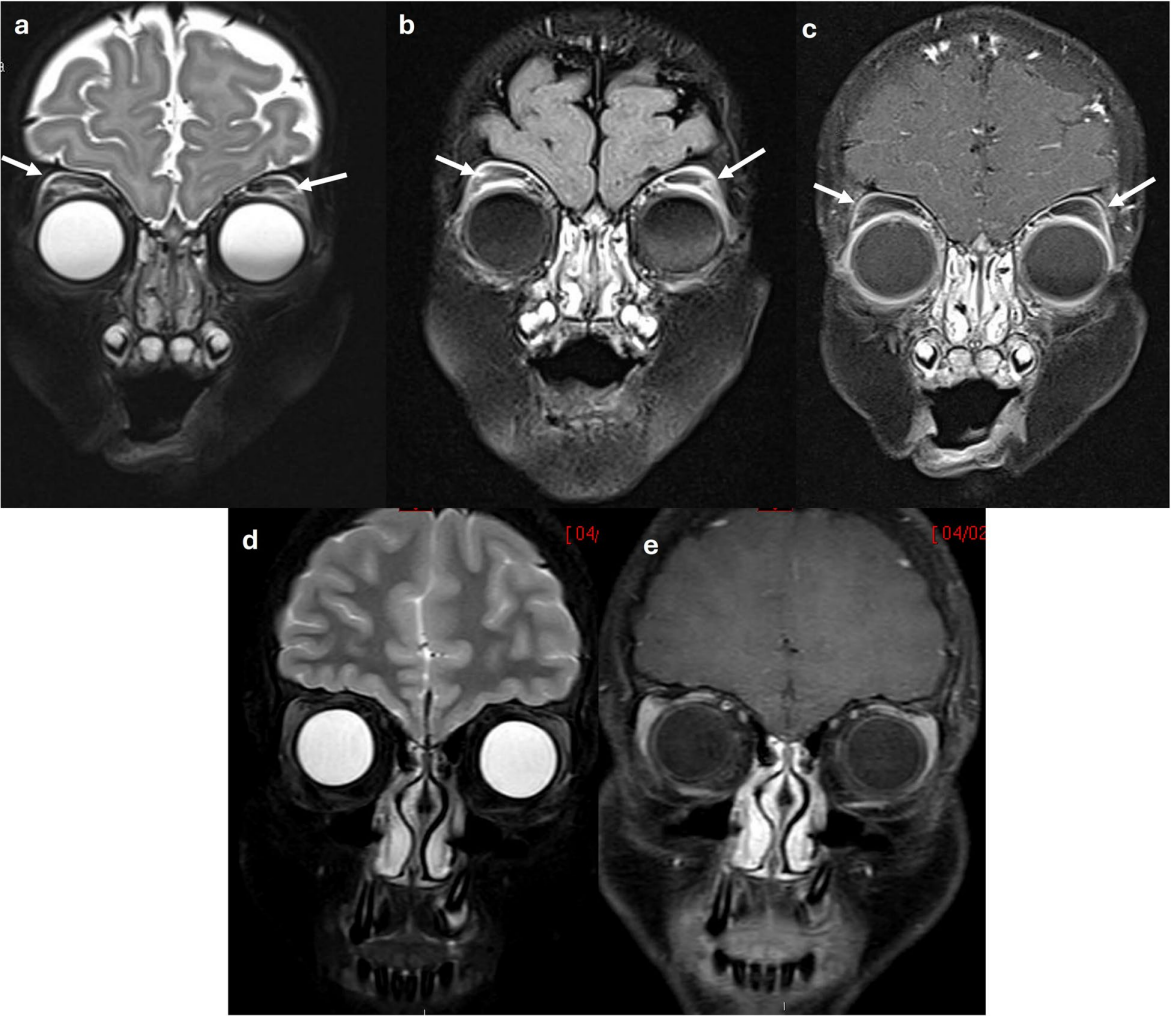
Images of a 7-month-old girl (a–c) and a 13-year-old girl (d–e). In the younger patient, linear T2 hyperintensity is present along the superior extraconal orbital fat on the fat-suppressed coronal T2-weighted image (a, *arrows*) and on the coronal T2-FLAIR image (b, *arrows*), with subtle corresponding linear enhancement on the coronal post-contrast T1-weighted image (c, *arrows*). The older patient demonstrates normal signal intensity of the superior extraconal orbital fat on the fat-suppressed coronal T2-weighted image (d) and on the coronal post-contrast T1-weighted image (e)
